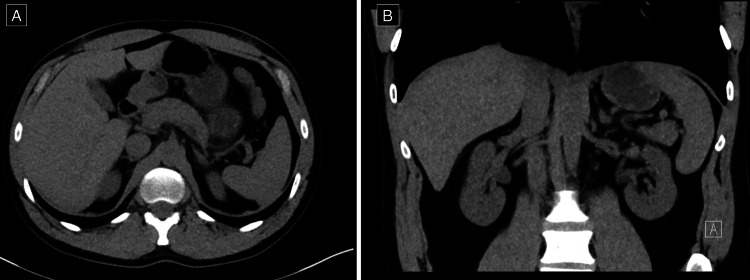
Computed tomography adrenals demonstrating complete resolution of the left adrenal haematoma on (A) axial view and (B) coronal view at five months follow-up.
